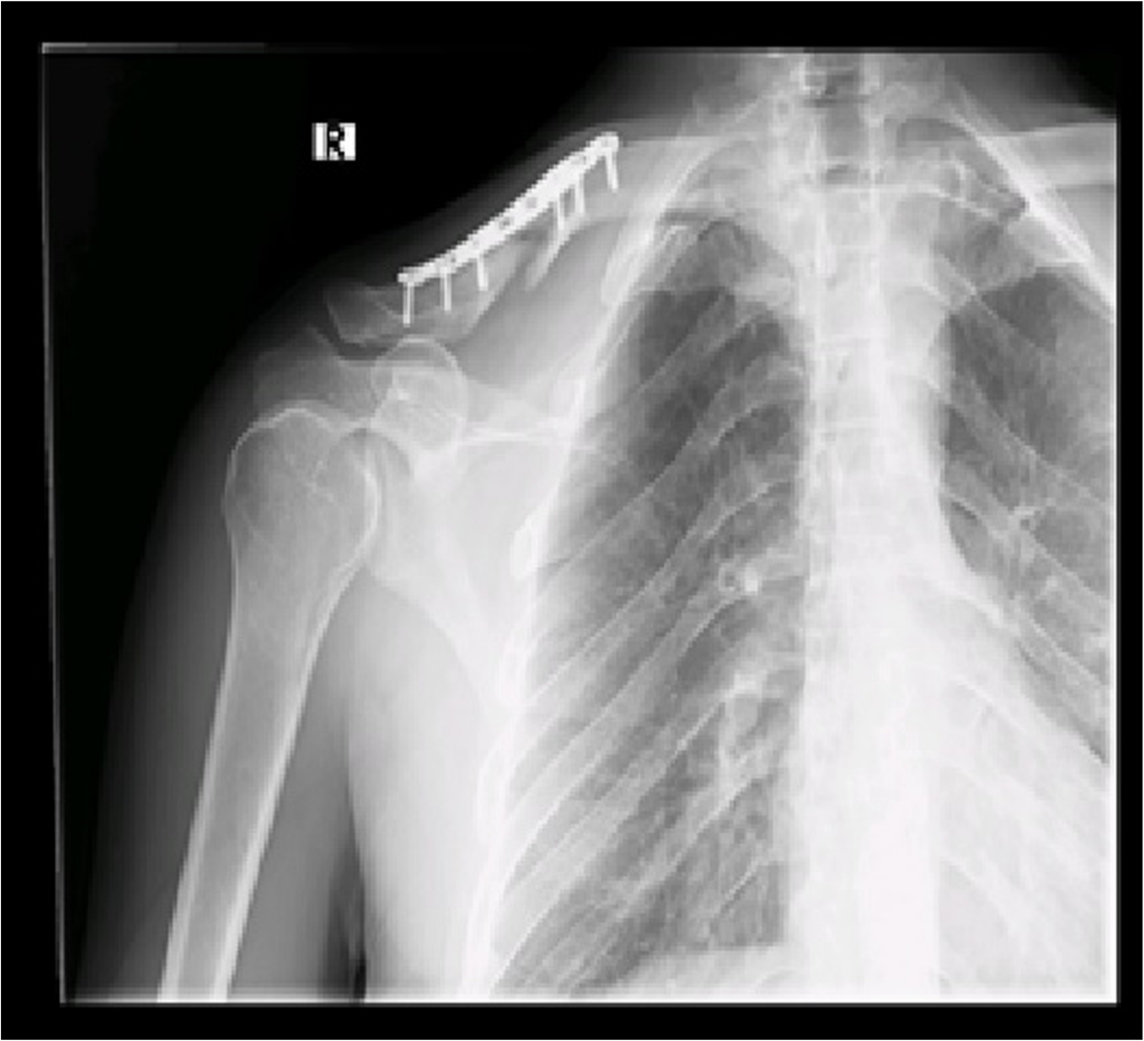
Postoperative 2-week follow-up X-ray
